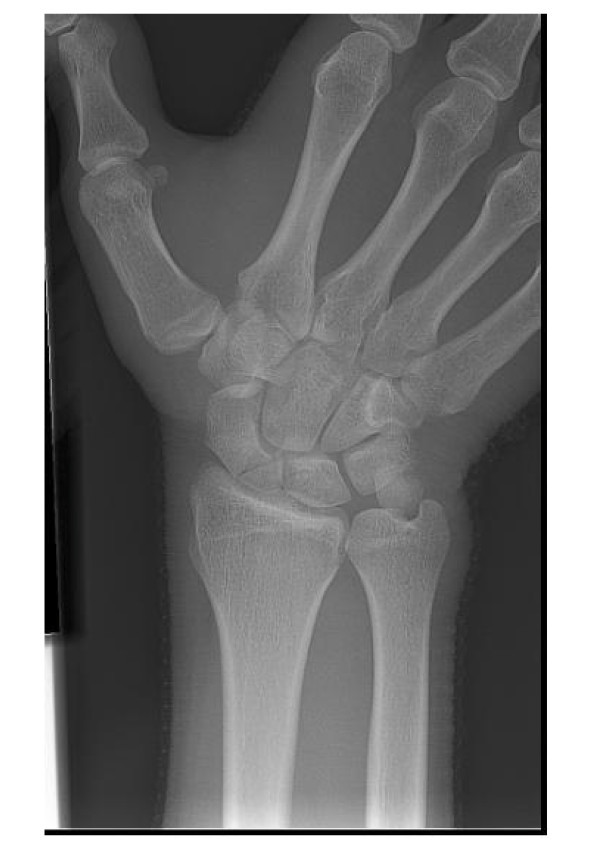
Proximal pole fracture (patient E) on a conventional radiograph.
